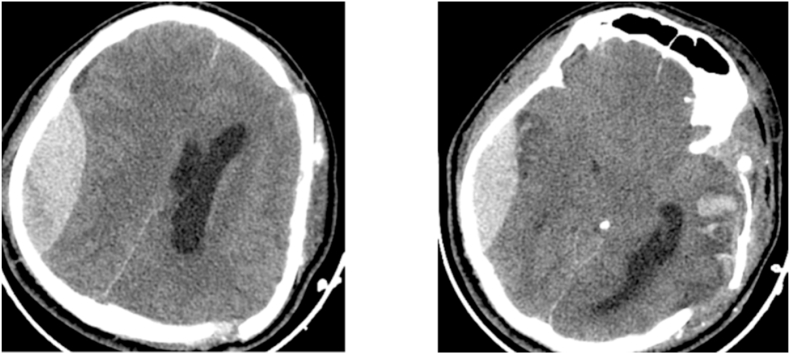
Post-operative CT scan showing a large right extradural hematoma with mass effect and decompressive craniectomy of left side.
